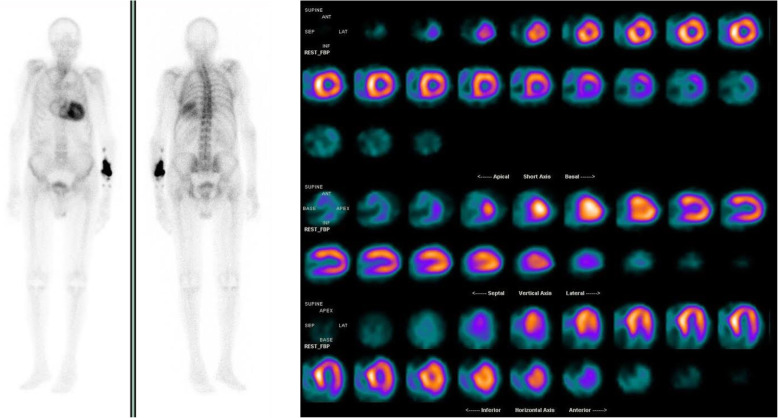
Bone tracer scintigraphy revealing high diffuse myocardial uptake (Perugini score 3)
